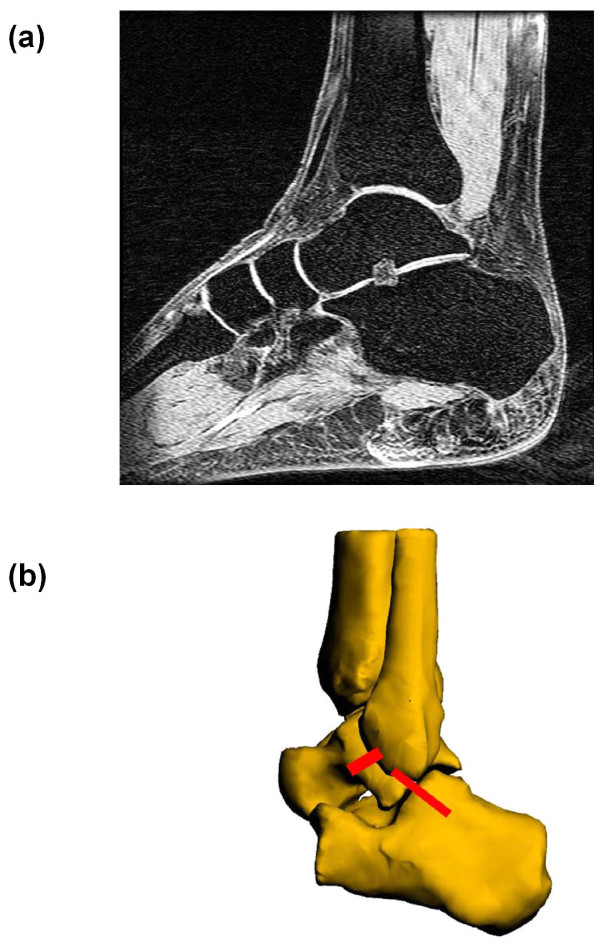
(a) MR image of the ankle joint complex (AJC) used to create a three-dimensional virtual model (b) 3D model of the AJC with anterior talofibular ligament (ATFL) and calcaneofibular ligament (CFL).
